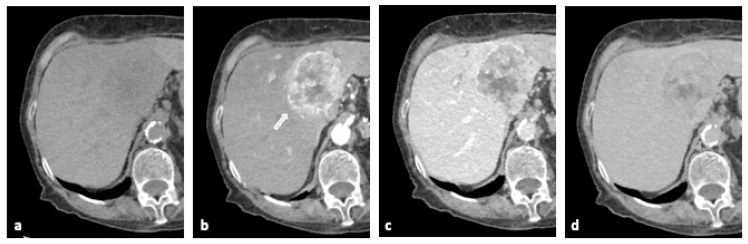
CE-CT features of iCCA. An example of the enhancement of iCCA from CE-CT: (a) unenhanced CT: hypodense mass. (b) The arterial phase shows peripheral border enhancement (arrow). (c) The portal venous phase and (d) the delayed phase show progressive centripetal enhancement. Abbreviations: iCCA, intrahepatic cholangiocarcinoma; CE-CT, contrast-enhanced computed tomography; CT, computed tomography.
